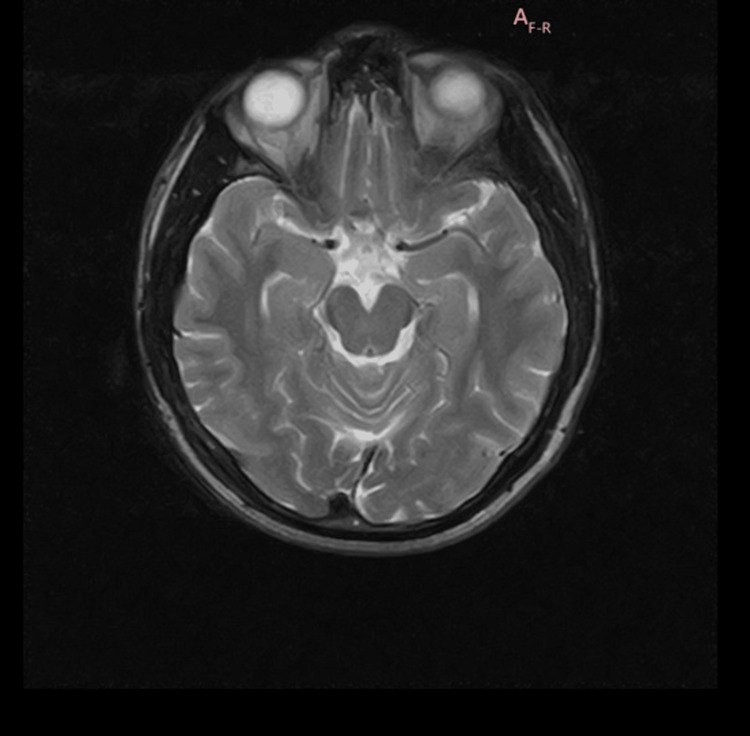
T2 sequence of MRI brain without contrast showing no abnormalities
